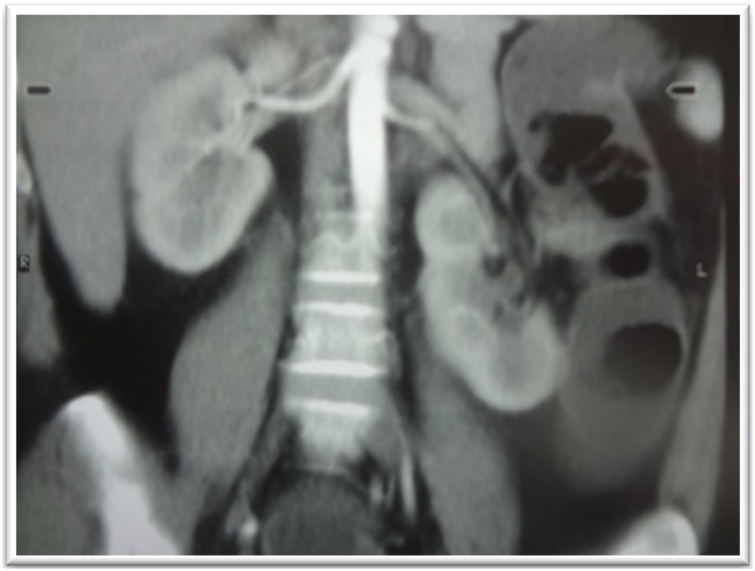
Abdominal CT urogram showing left renal malroatation and left adrenal mass (case 2).
